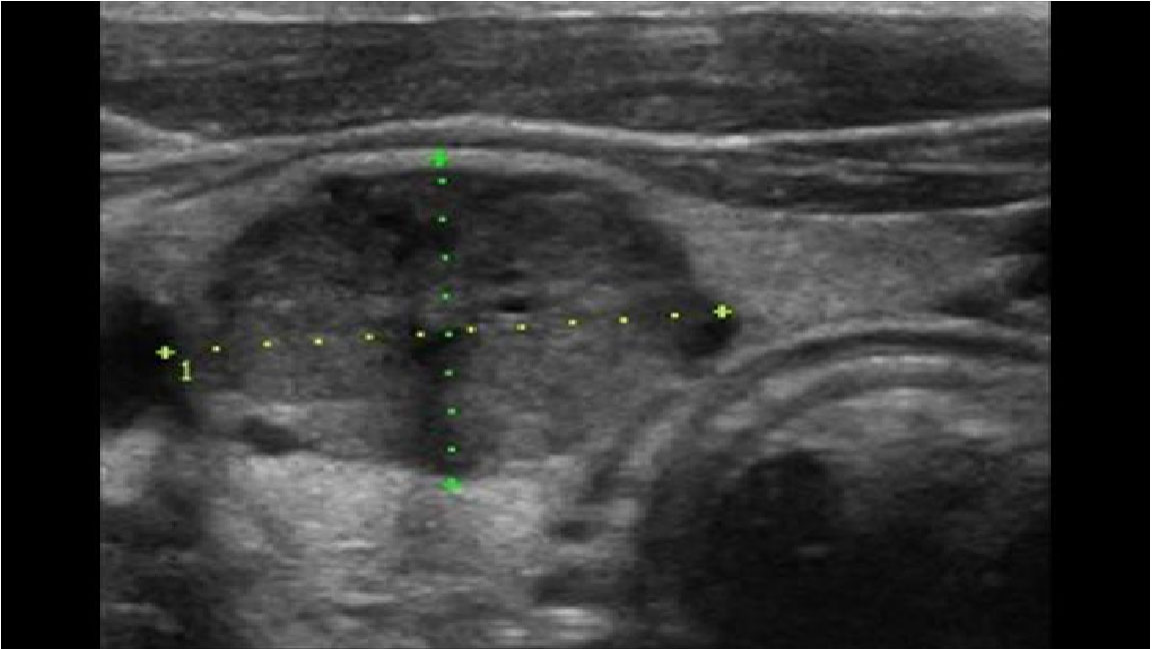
MTC in ultrasound: solid inner content, ovoid to round, hypoechoic (50%), calcifications (50–80%).
